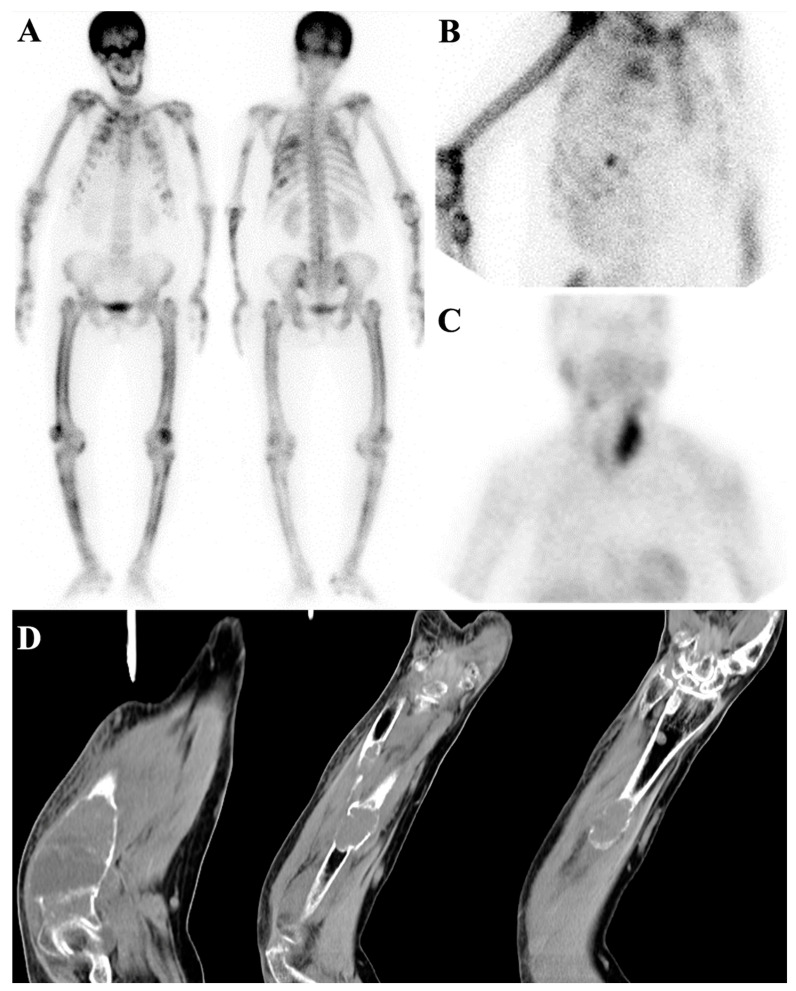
Atypical case of metabolic superscan in a patient with a history of generalized bone pain and recent swelling of the right distal humerus and proximal radius (A). Upon examination, the bone scan reveals bone expansion and a focal rib uptake consistent with a fracture (B). Parathyroid scintigraphy uncovers a sizable ^99m^Tc-sestamibi-avid lesion on the left side, suggestive of parathyroid adenoma/carcinoma, which is later confirmed to be parathyroid carcinoma (C). Correlative CT images exhibit lytic-destructive lesions with bone expansion in the right radius, indicating a probable brown tumor (D). Visualization of the kidneys in the bone scan may imply a rapidly progressive nature of the disease and good functioning kidneys in a previously healthy middle-aged woman.
